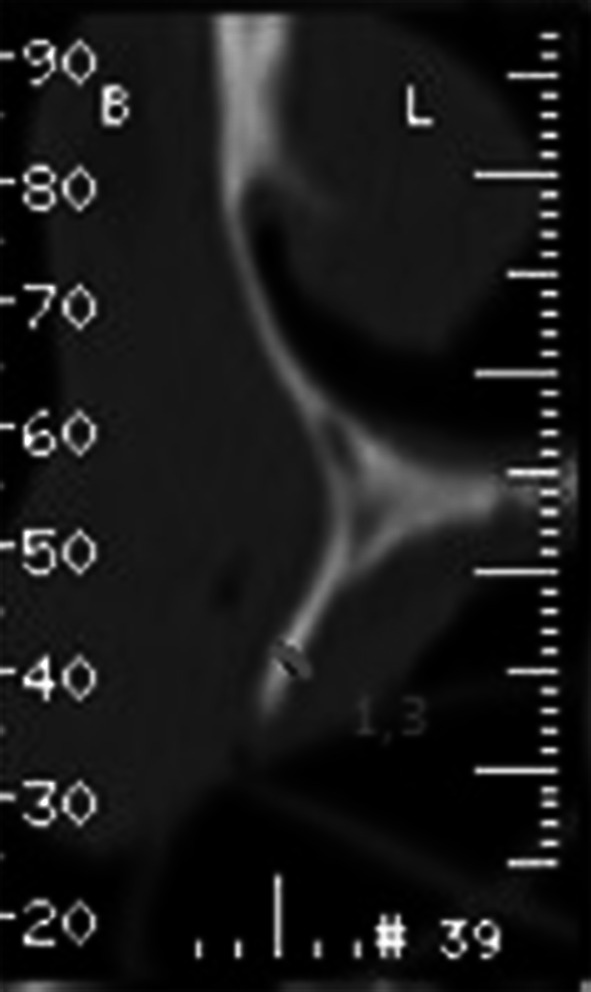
CT before grafting within location of tooth 16
